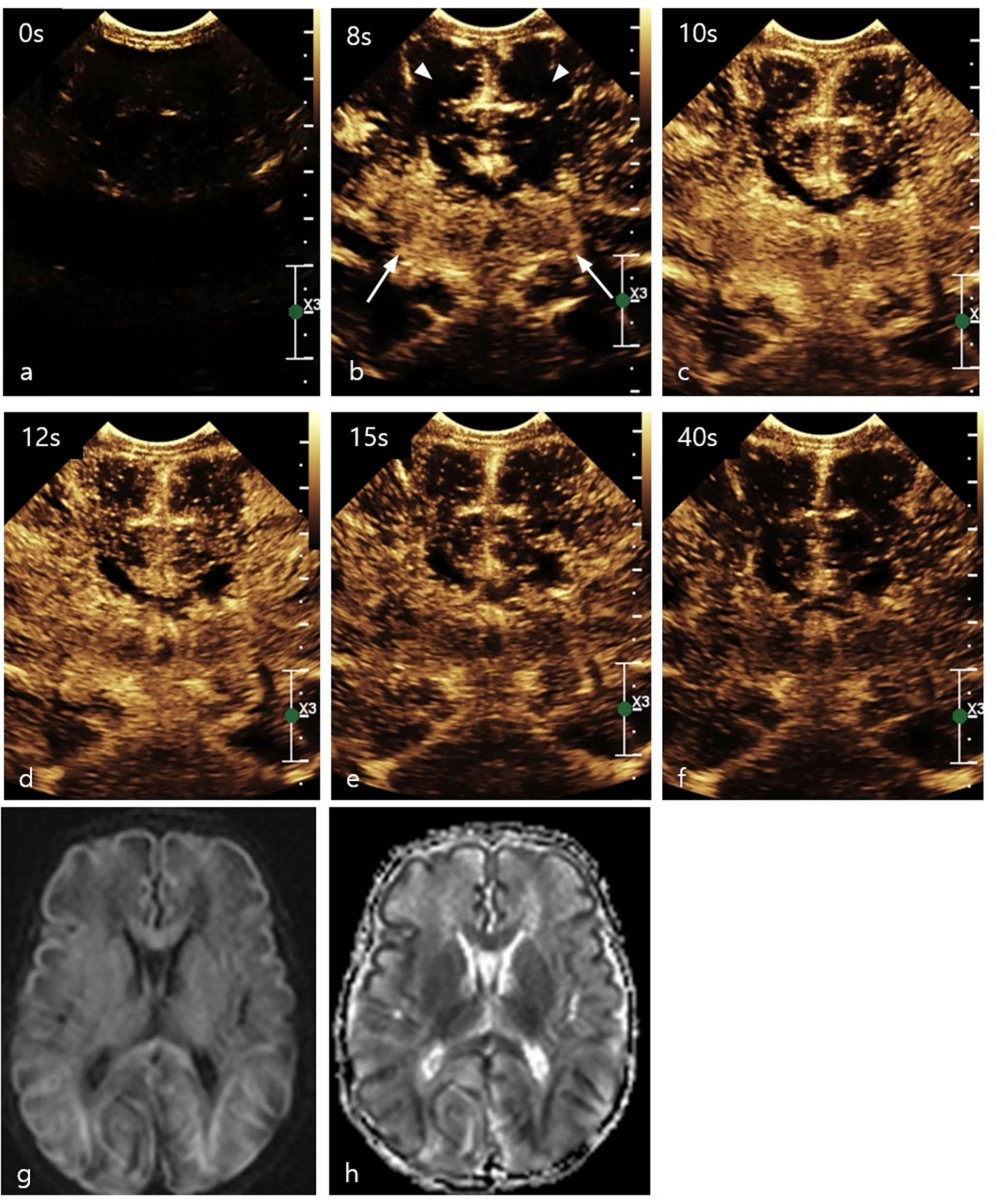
Brain contrast-enhanced ultrasound (CEUS) and MRI scans of hypoxic-ischemic injury in a 21-day-old boy. (a) Mid-coronal brain scan before the second contrast administration, with a few residual microbubbles from the first injection. (b) Non-homogeneous enhancement in the basal ganglia (arrows) with a paucity of microbubbles in the cortex (arrowheads) is noted at 8 s. (c) The same plane at 10 s post-contrast injection demonstrates progressive non-homogeneous enhancement in the basal ganglia and minimal fill-in of contrast in the cortex at 10 s. More avid enhancement of the cortical sulci/leptomeninges as compared to the cortex is noted. (d) The same plane at 12 s post-contrast injection demonstrates subtle wash-out of contrast in the basal ganglia, while minimal progressive fill-in of contrast in the cortical gyri more than in the cortex. (e) The same plane at 15 s post-contrast injection demonstrates progressive wash-out of contrast from both the cortex and the basal ganglia. (f) The same plane at 40 s post-contrast injection demonstrates moderate wash-out of contrast from the cortex and the basal ganglia. (g) Diffuse signal abnormalities in the cortical ribbon, white matter, and basal ganglia are noted in diffusion-weighted imaging (DWI) of the mid-axial brain. (h) The corresponding apparent diffusion coefficient map demonstrates low signal intensities in the regions affected in the DWI. Republished from *Hwang M*,* Zhang Z*,* Katz J et al. (2022) Brain contrast-enhanced ultrasonography and elastography in infants. Ultrasonography 41(4):633–649.* 10.14366/usg.21224
